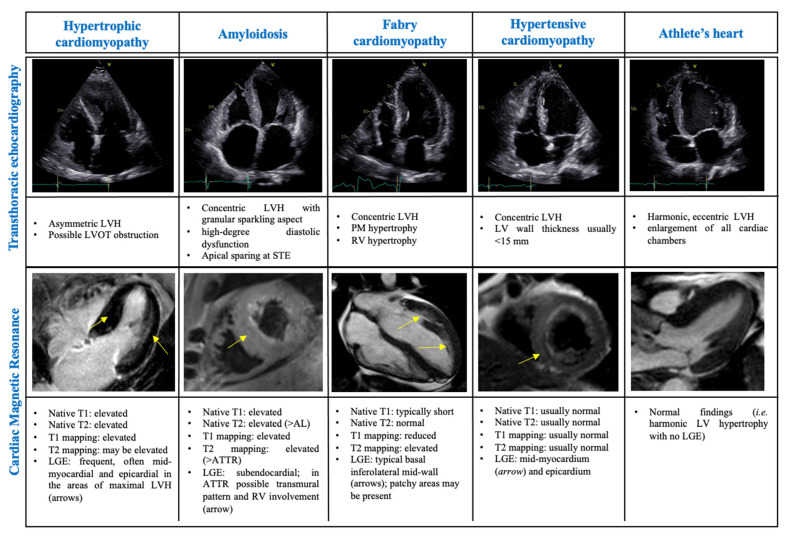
Echocardiographic and cardiac magnetic resonance ‘red flag features’ for the diagnosis of hypertrophic cardiomyopathy and phenocopies. Abbreviations: AL, light-chain amyloidosis; ATTR, transthyretin amyloidosis; LGE, late gadolinium enhancement; LV, left ventricle; LVH, left ventricular hypertrophy; LVOT, left ventricular outflow tract; RV, right ventricle, STE, speckle tracking echocardiography.
